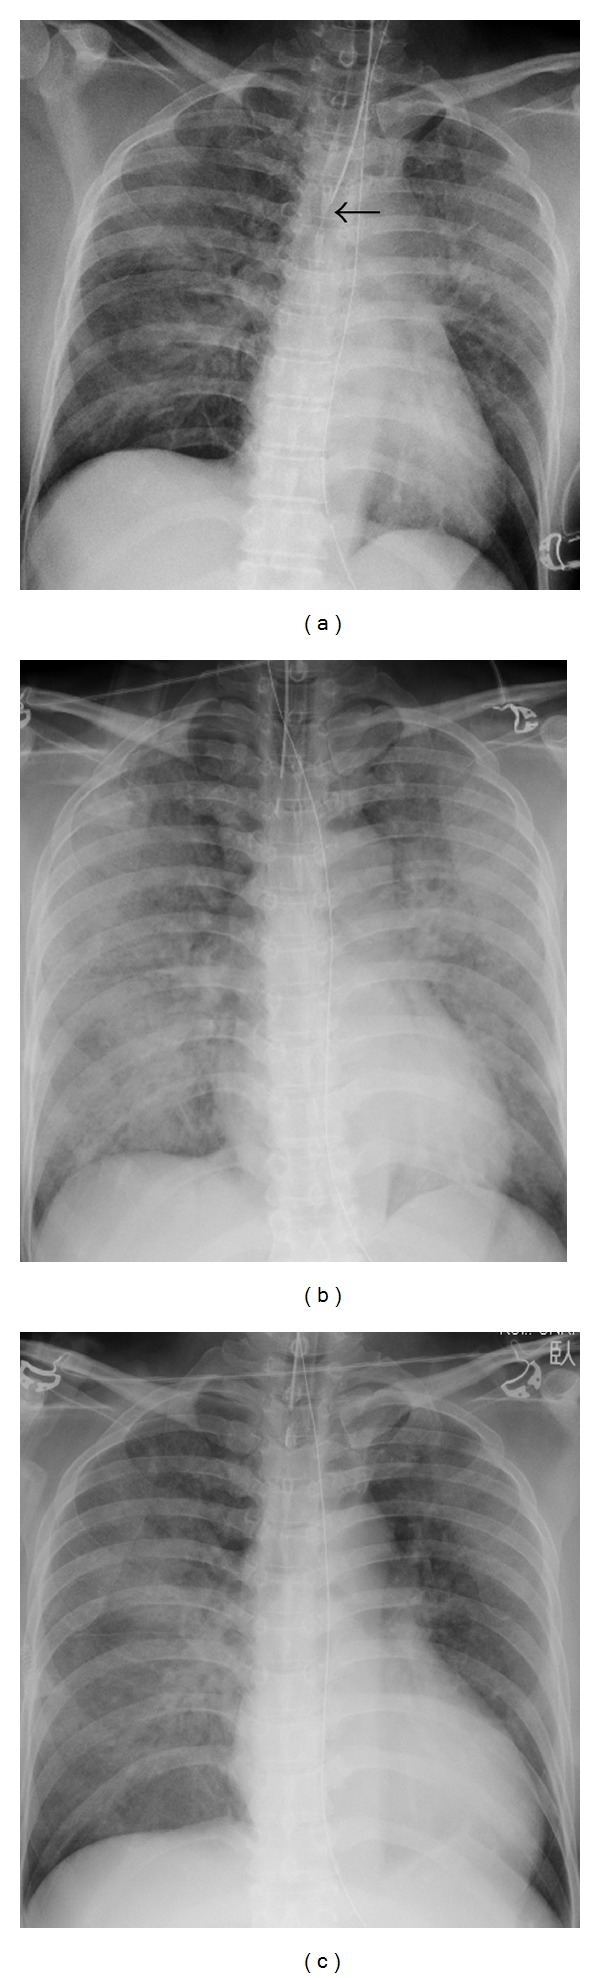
(a) A postoperative chest radiograph taken in the operating room showed bilateral diffuse ground glass attenuation. The central shadow was not widened: the cardiopulmonary rate was 48%. The tip of the intratracheal tube was located near the tracheal bifurcation (black arrow). (b) A chest radiograph demonstrated progression of the diffuse ground glass attenuation and appearance of alveolar consolidation. The photograph was taken in the intensive care unit 2 hours after surgery. (c) A chest radiograph revealed significant resolution of pulmonary abnormalities 3 days after the operation.
